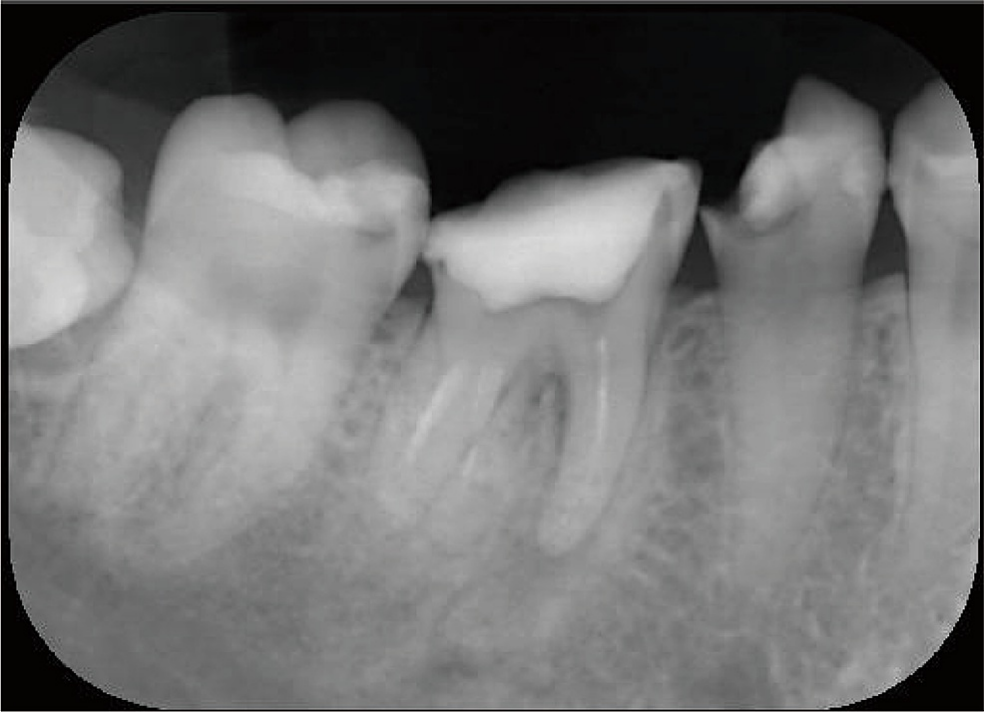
Dental radiograph of #37.
